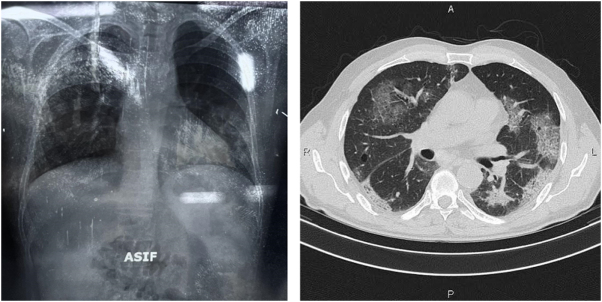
The chest X-ray shows bilateral infiltration.
